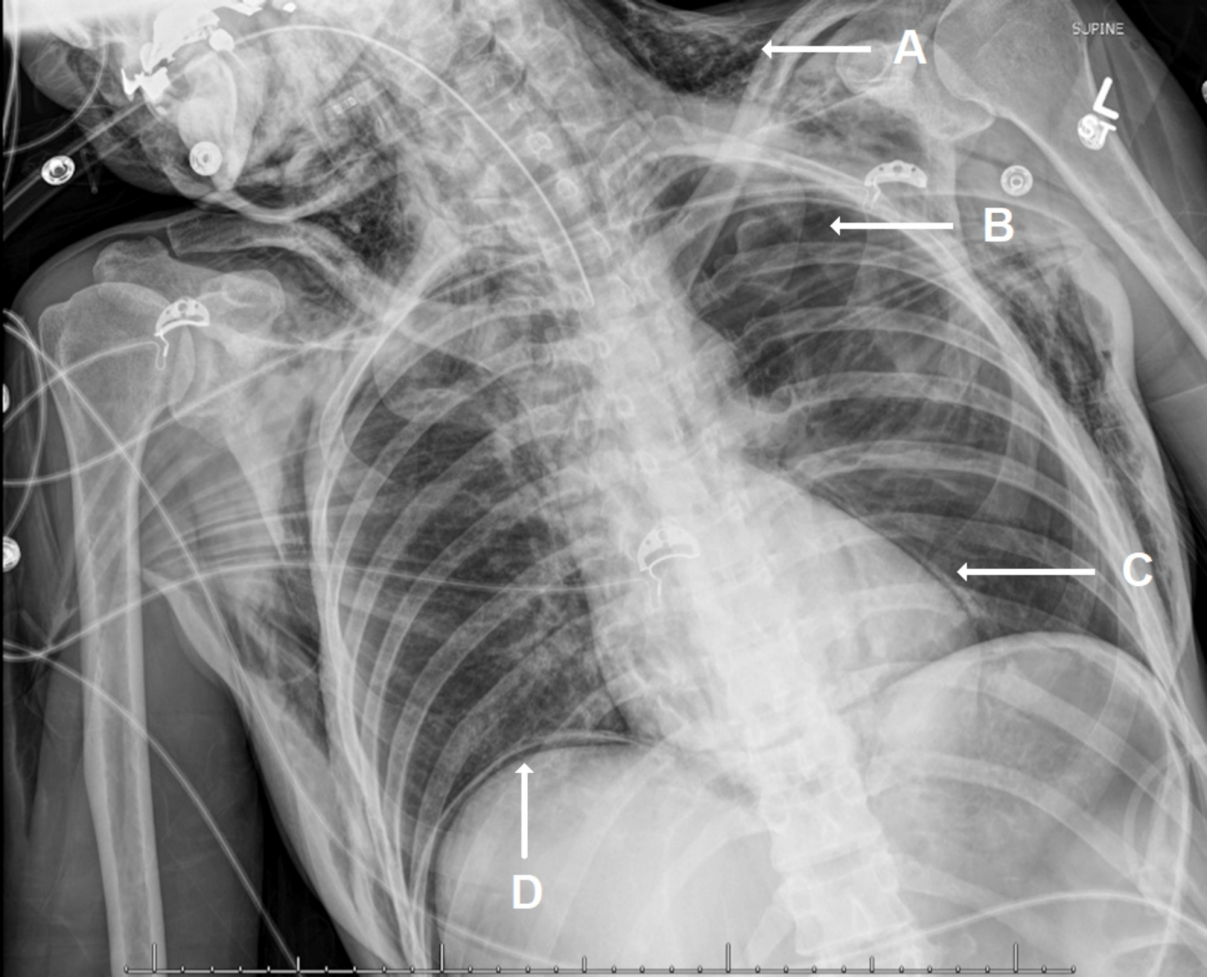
Chest Radiograph Anterior-posterior chest radiograph depicting diffuse subcutaneous emphysema (A), pneumothorax (B), pneumomediastinum (C), and subdiaphragmatic air (D) (diaphragm sign).
